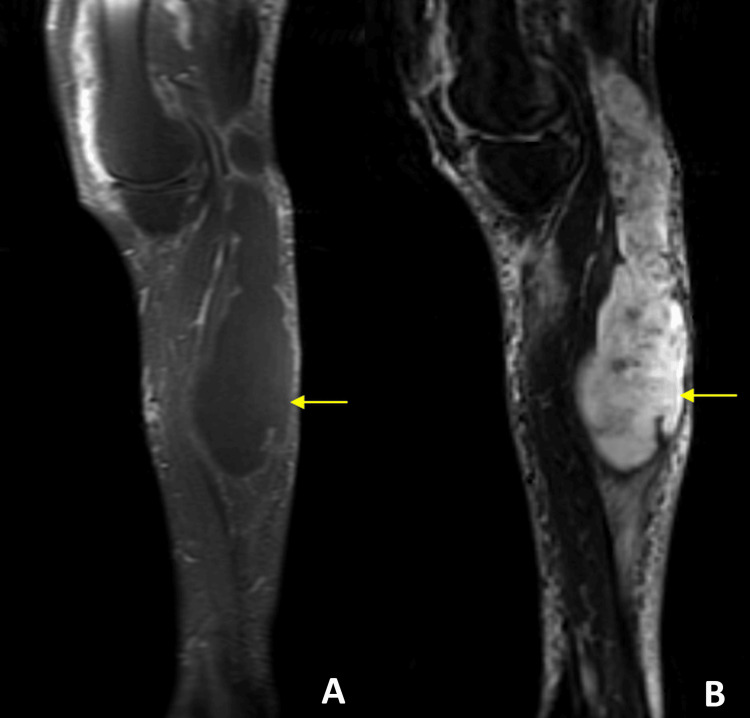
Sagittal MRI demonstrating a giant Baker’s cyst (yellow arrows) with mass effect. (A) The sagittal T1-weighted post-contrast image shows the cyst as a well-defined lesion with relatively homogeneous signal intensity. (B) The sagittal STIR image demonstrates the cyst with characteristic hyperintense signal consistent with fluid content. STIR: Short Tau Inversion Recovery
